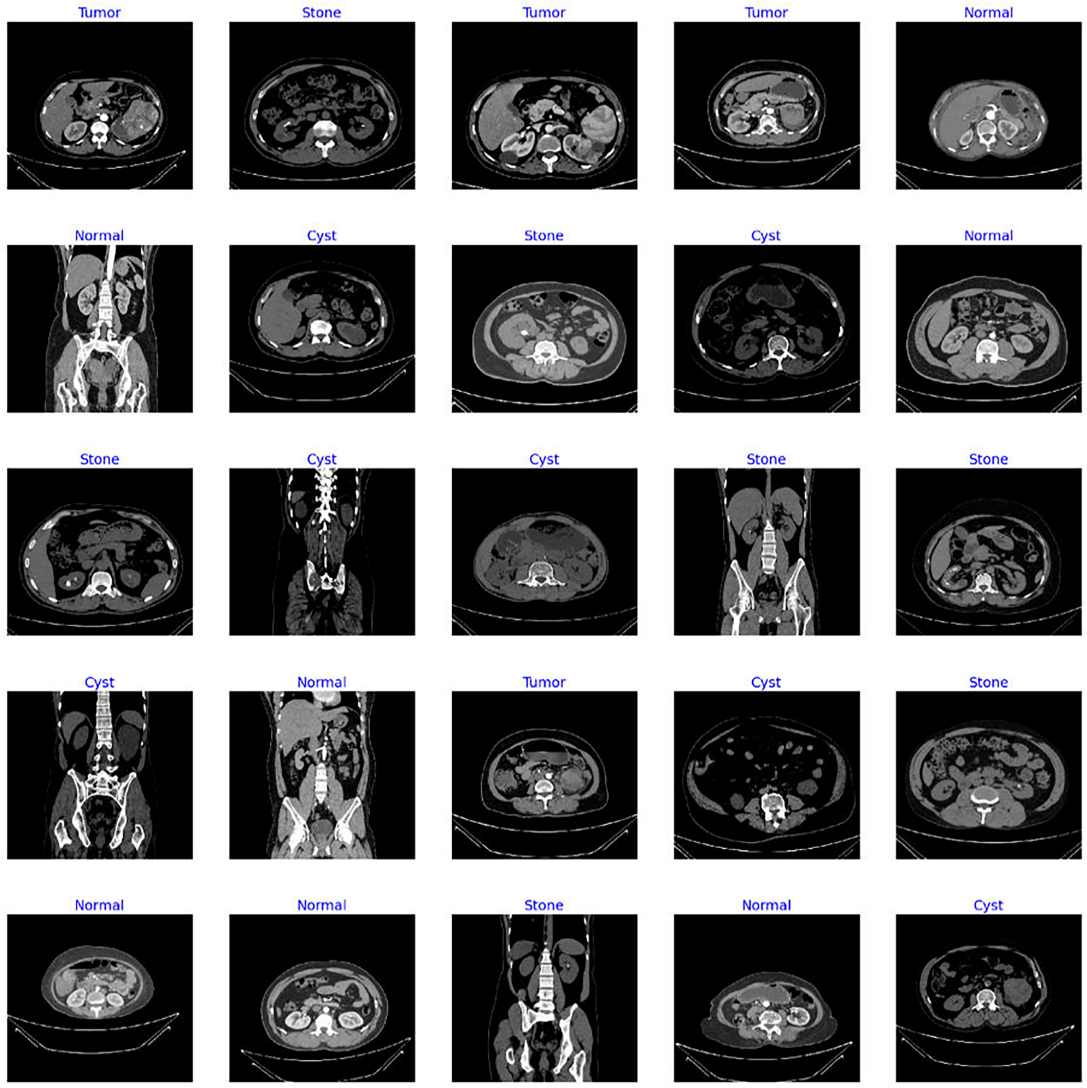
Sample images from standard CT kidney database.
